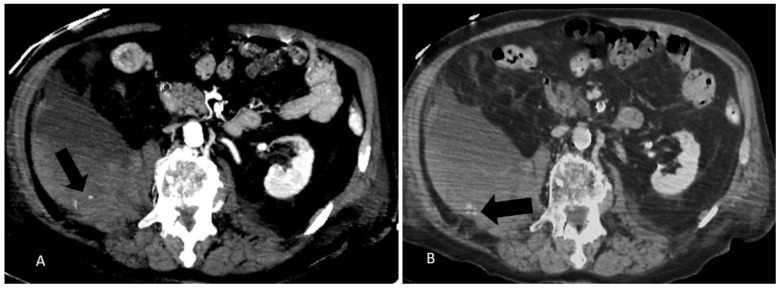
(A) Arterial phase CT and (B) late phase CT of a right retroperitoneal and ileo-psoas hematoma. Note multiple arterial intralesional blushes (arrow in (A)) show a contrast late enhancement (arrow in (B)).
